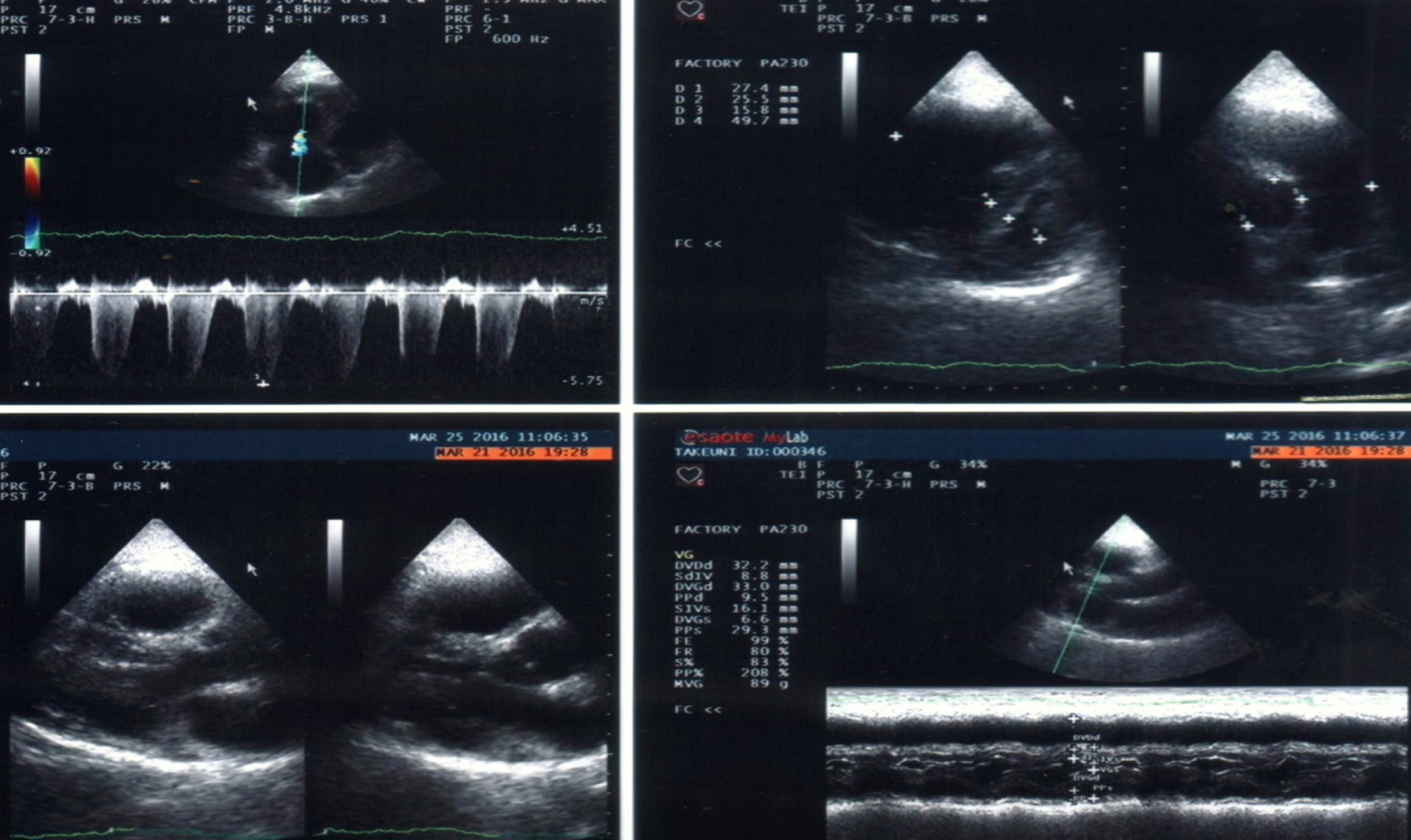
Echocardiogram of case number 2 shows markedly dilated right cavities with marked mass effect on the left cavities. The pulmonary systolic pressure was estimated at 148 mmHg.
